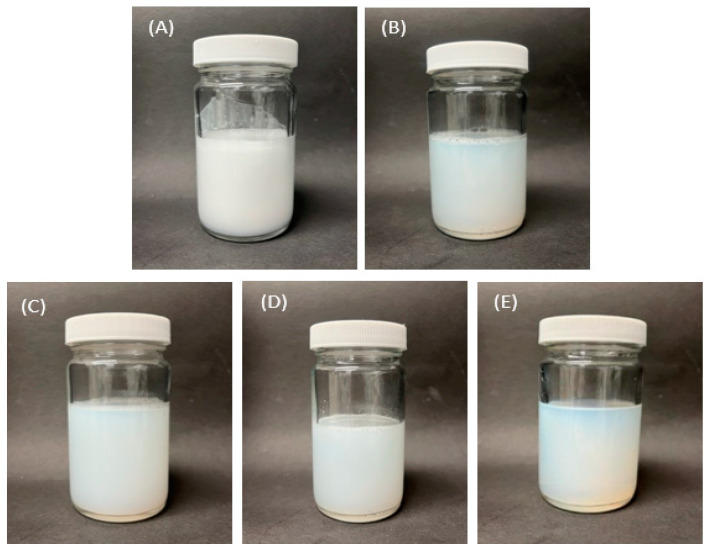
Liposome solutions prepared using (A) homo mixer, (B) microfluidizer, (C) bath sonication, (D) horn sonication, and (E) focused ultrasonication.
